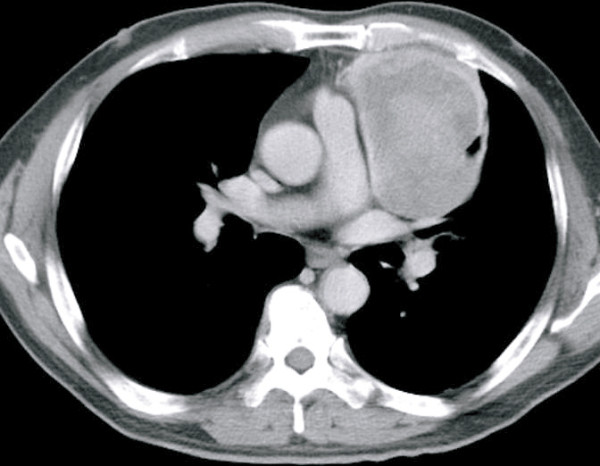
Enhanced chest computed tomography shows a heterogeneous 9.0 × 7.5 cm mass arising in the anterior mediastinum.
